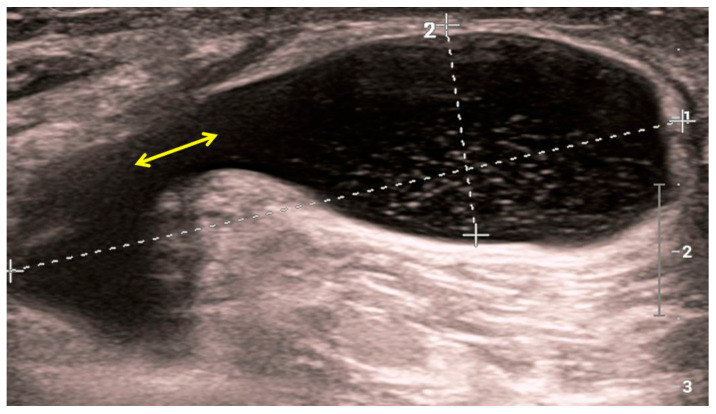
Sonographic image of a Baker’s cyst in the medial part of the popliteal fossa. Punctiform internal echoes from the cyst lumen which communicate with the joint space of the knee joint (yellow bidirectional arrow). Fluid within the cyst enhances the ultrasound beam posteriorly.
